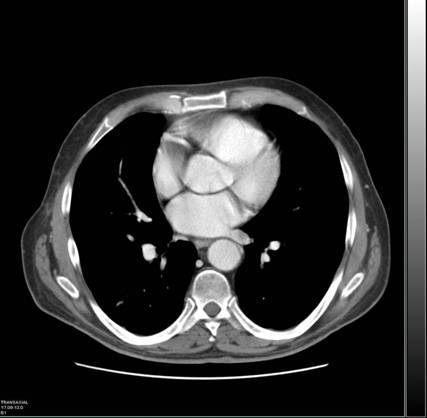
First CT scan performed in which no atrial lesion was reported.
